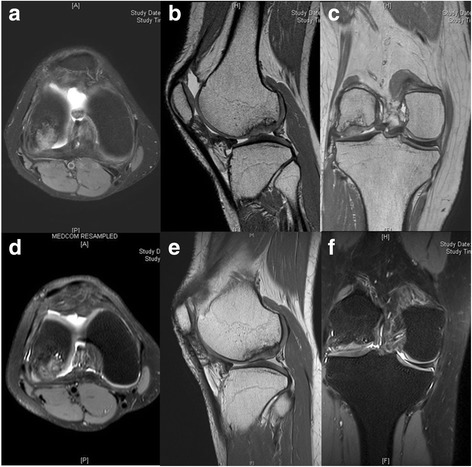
Magnetic resonance image. a, b, c The repair of the osteochondral defect at postoperative 1 year was observed and d, e, f the repaired tissue was maintained for 5.5 years without deterioration
